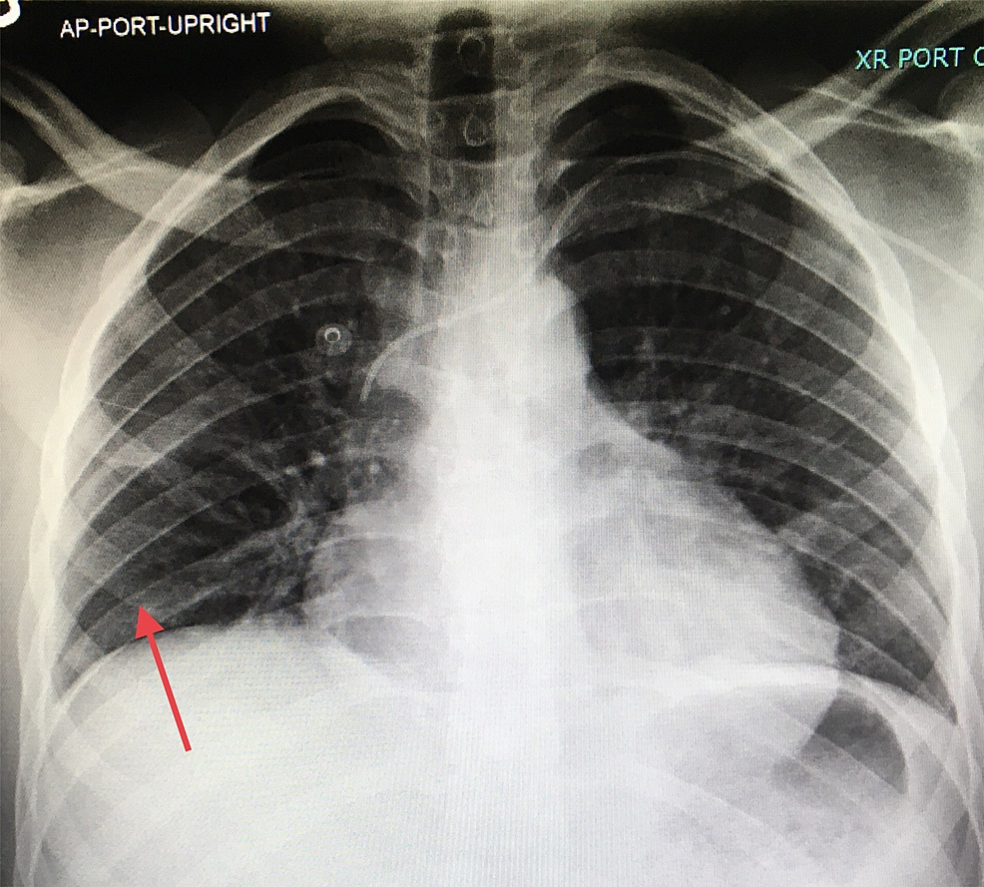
Chest X-ray Following chest tube removal, no hemothorax or pneumothorax was seen in the right chest (red arrow).
